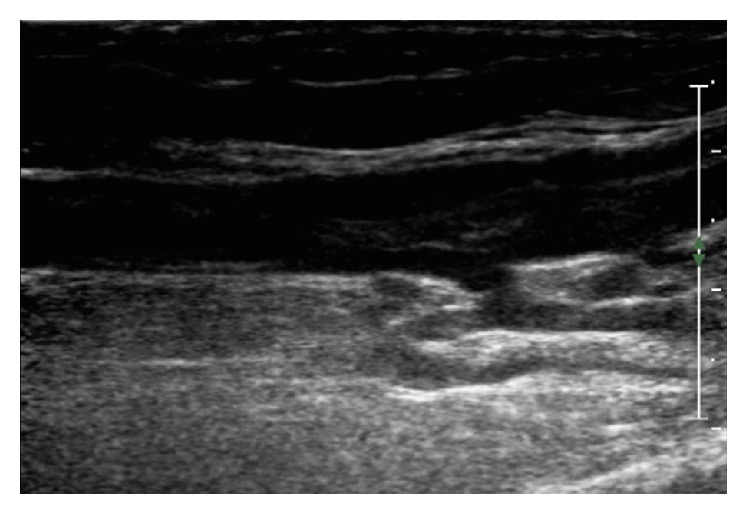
Right upper extremity ultrasound of a 67-year-old man revealing acute venous thrombosis in the brachial vein and peripheral axillary vein with acute superficial thrombosis in the right basilica vein.
